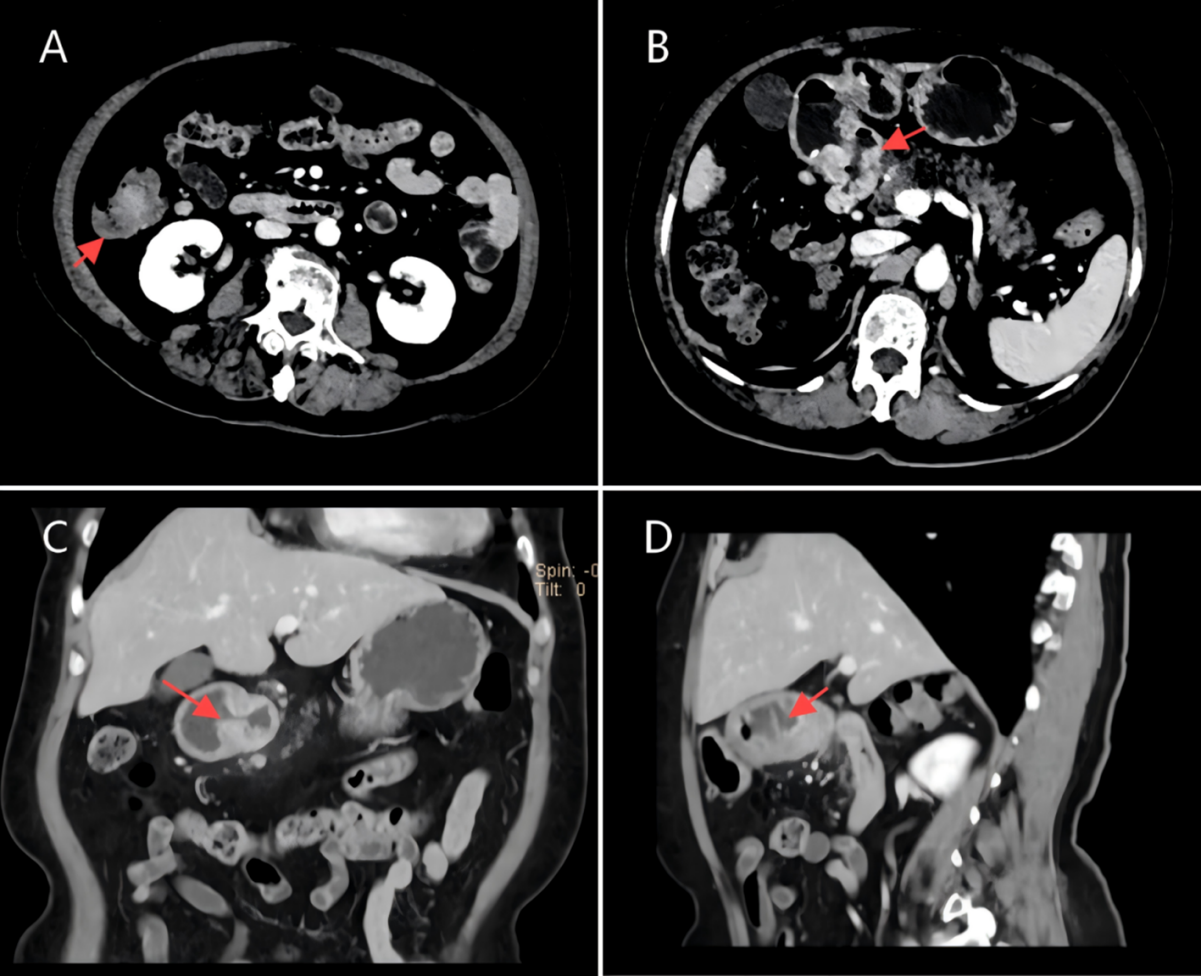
(A,D) CT scan image of a tumor in the ascending colon, dated April 7, 2023. (B,C) CT scan image of a tumor in the duodenal bulb, dated April 7, 2023.
